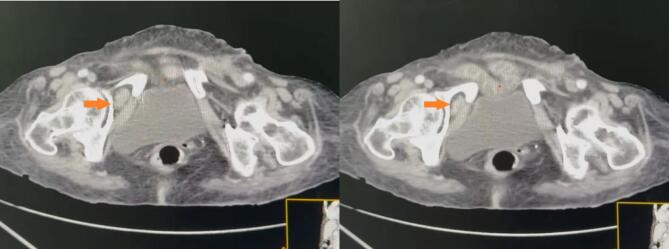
A, B: Axial CT scan showing a right-sided obturator hernia.
